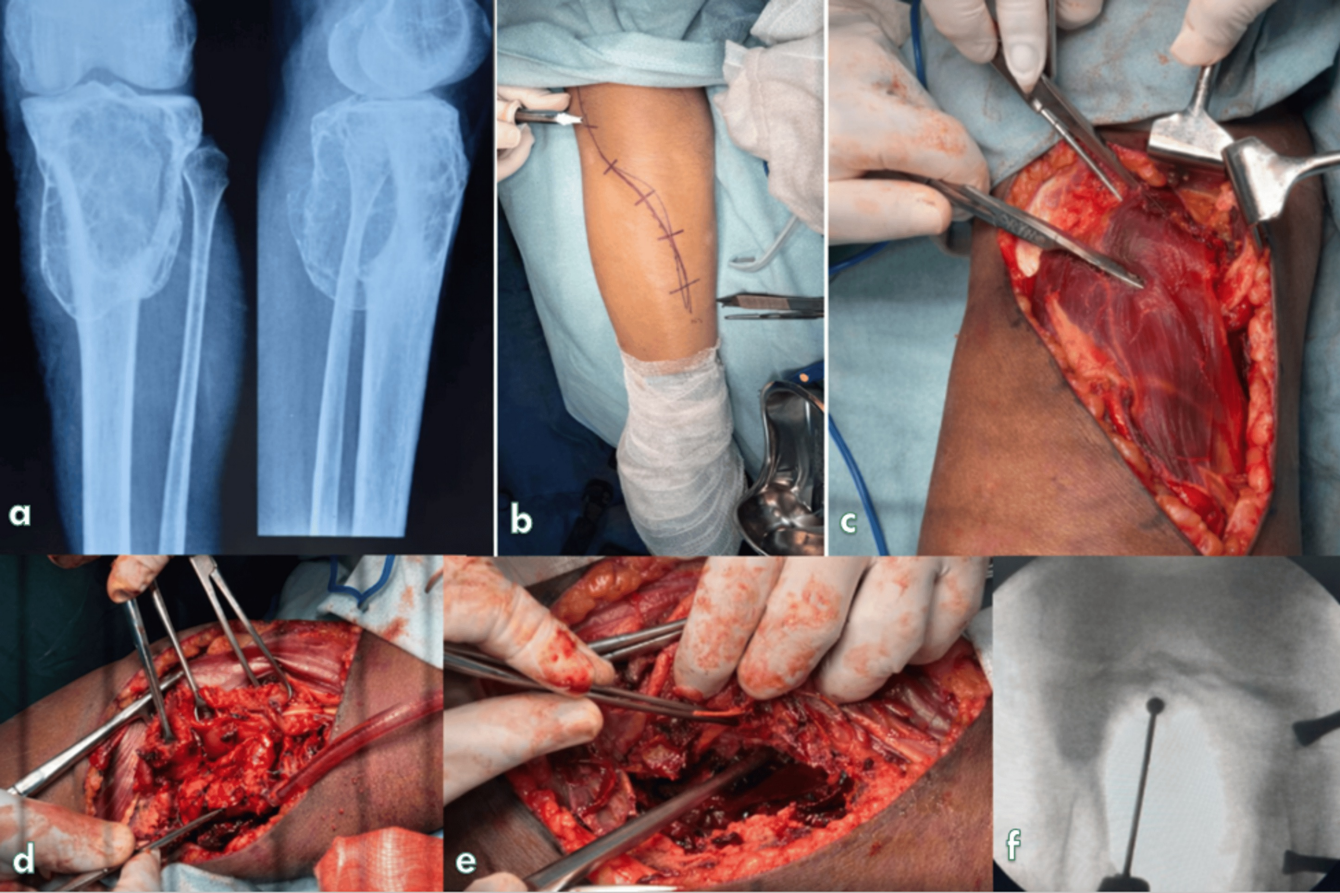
Representative preoperative radiographs and intraoperative surgical steps from patients in the present study demonstrating the direct posterior approach (a) Preoperative radiographs showing the involved knee.
